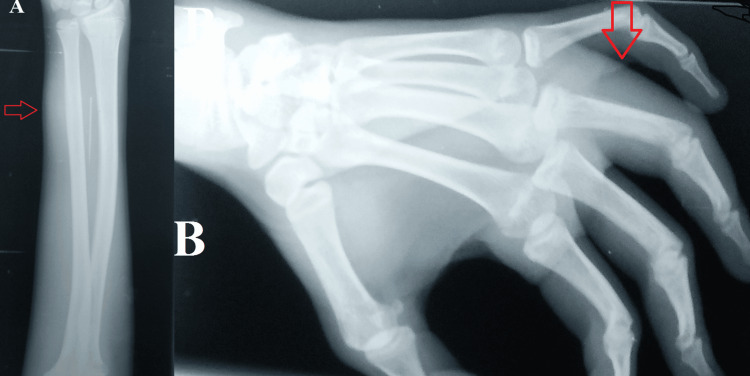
The left hand's plain radiographs. (A) Anteroposterior view showing the soft tissue swelling. (B) Oblique view showing the swollen ring finger.
